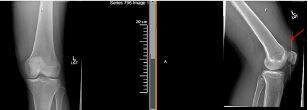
Radiograph of the left knee demonstrating a moderately sized effusion (arrow) without foreign body in a patient whose knee was punctured by an Agave plant spine.
